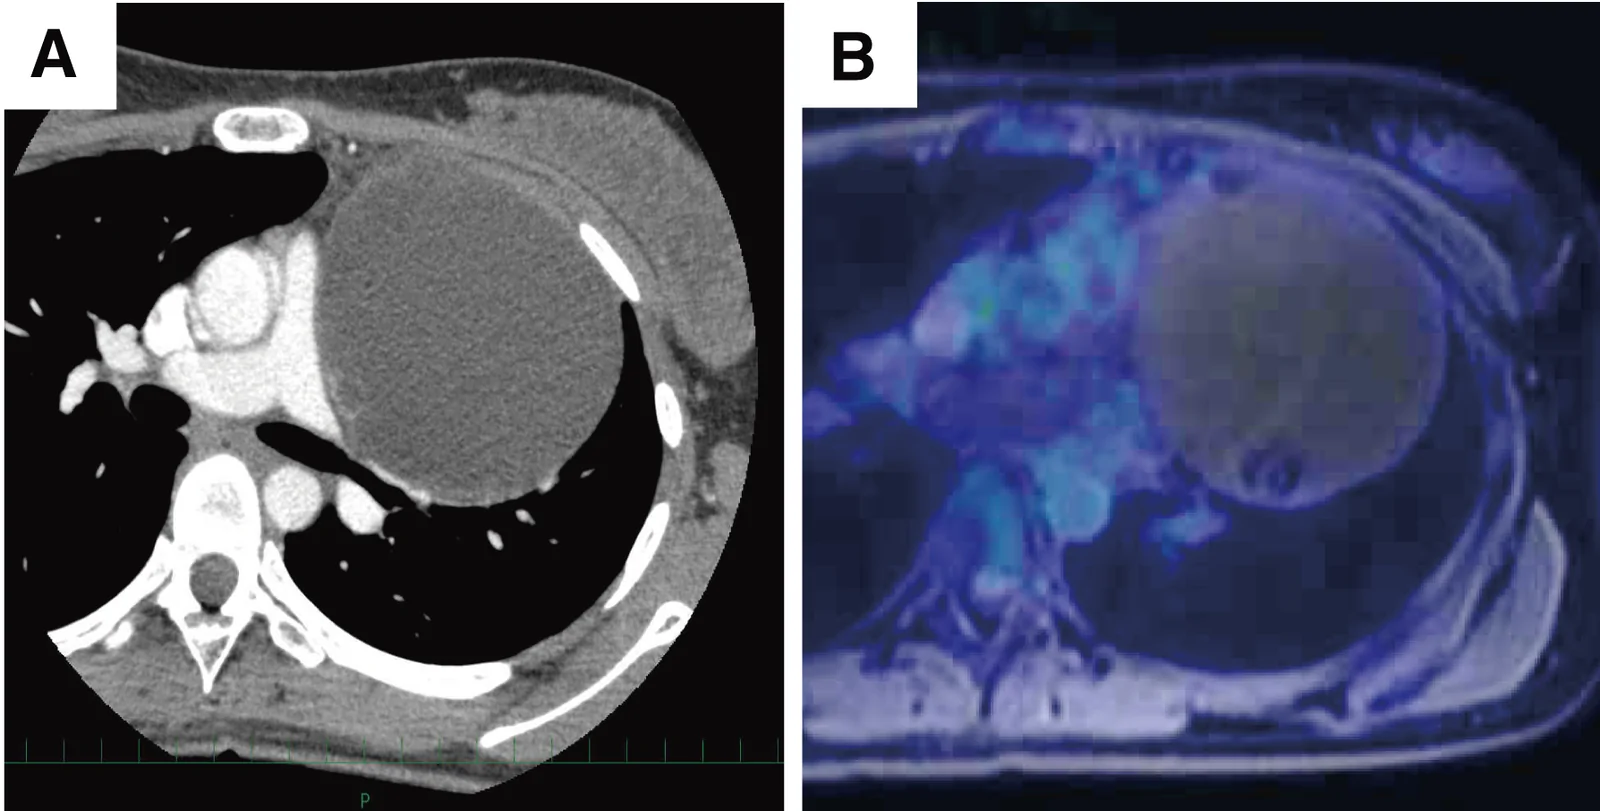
(A) Contrast-enhanced chest computed tomography showing an 8.6-cm predominantly cystic anterior mediastinal mass with fat components and minimal enhancement, protruding into the left thoracic cavity and compressing adjacent structures. (B) ^18^F-fluorodeoxyglucose positron emission tomography showing no significant uptake, consistent with a benign lesion.
